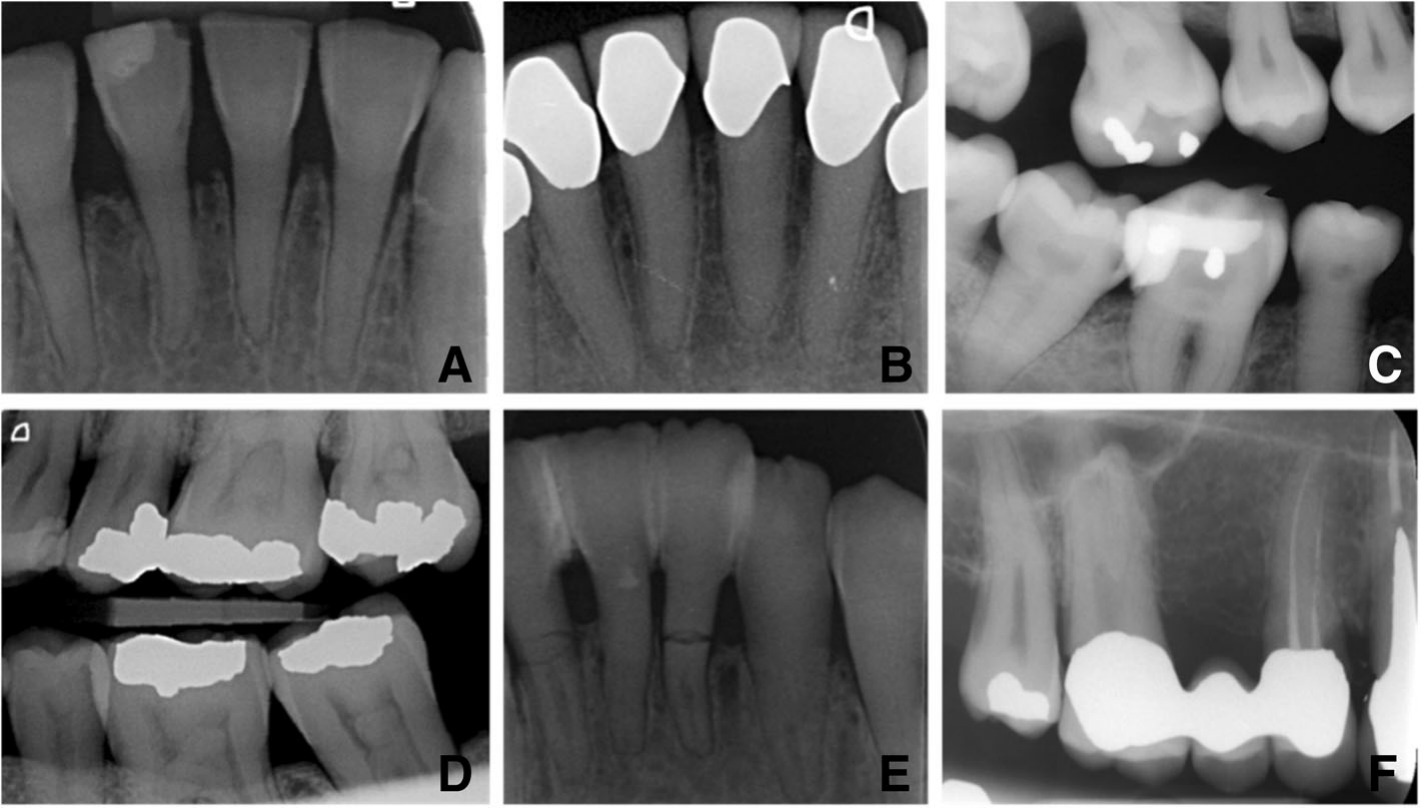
The variation of radiographic signs of DI as seen on dental radiographs of patients with OI. a OI type IV with DI, incisor with short root and pulp obliteration. b OI type IV with DI, incisor with pulp obliteration and treated with artificial crowns. c OI type III with DI, molars and premolars with cervical constriction. d OI type I, pulp stones in 26, 27, 36 and 37. e OI type III with DI, incisor with pulp obliteration and root fractures. f OI type I, taurodontism
